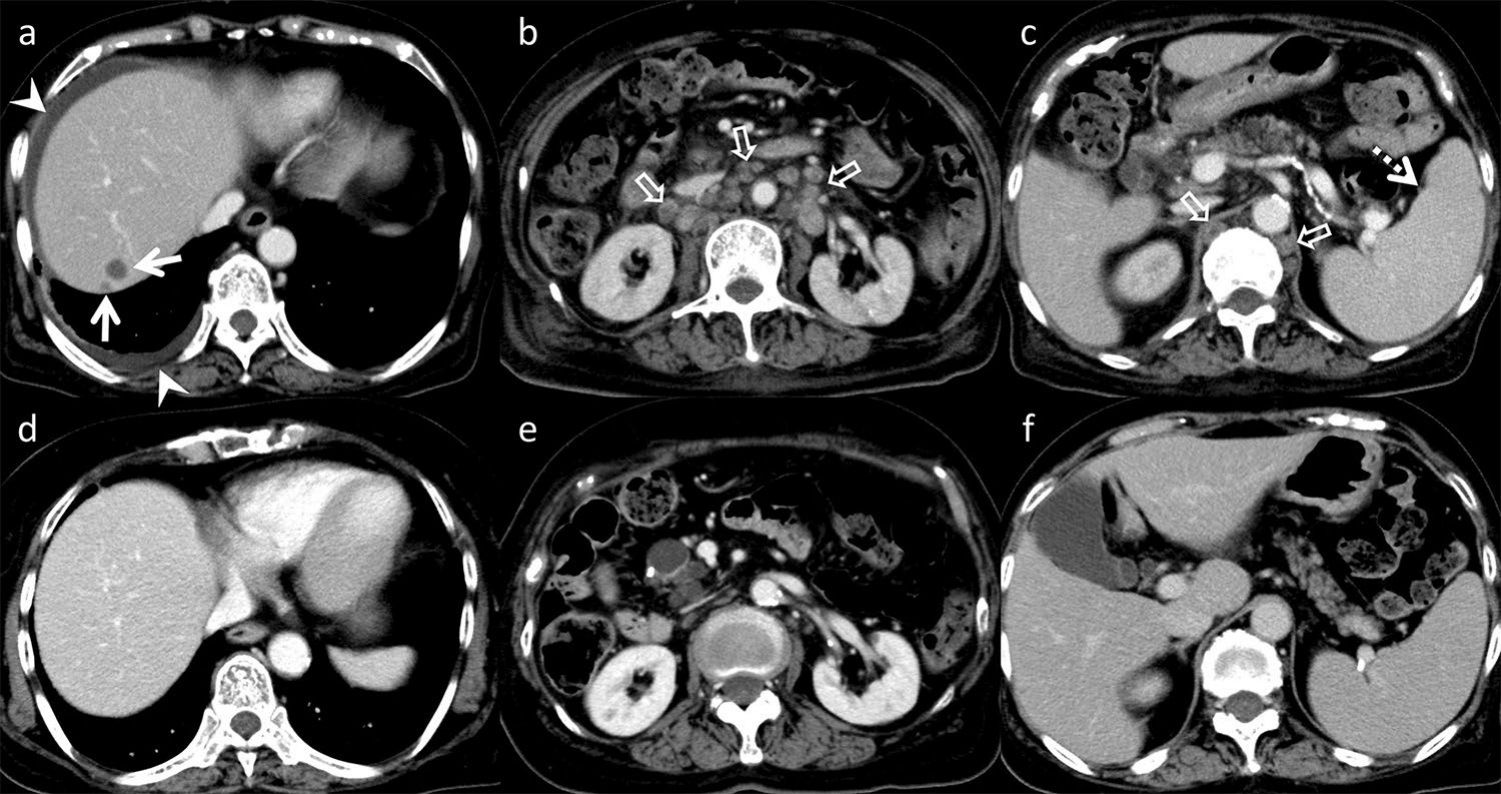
Methotrexate-associated lymphoproliferative disorder in a febrile 75-year-old man, who was taking methotrexate for rheumatoid arthritis. a–c Hypovascular hepatic nodules in S7 (arrows), ascites and right pleural effusion (arrowhead), lymphadenopathy (open arrows), and splenomegaly (dotted arrow) are observed on initial contrast-enhanced computed tomography (upper row). d–f These findings subsided four months after the withdrawal of methotrexate (lower row). It is difficult to differentiate methotrexate-associated lymphoproliferative disorder from malignant lymphoma on a single image alone
